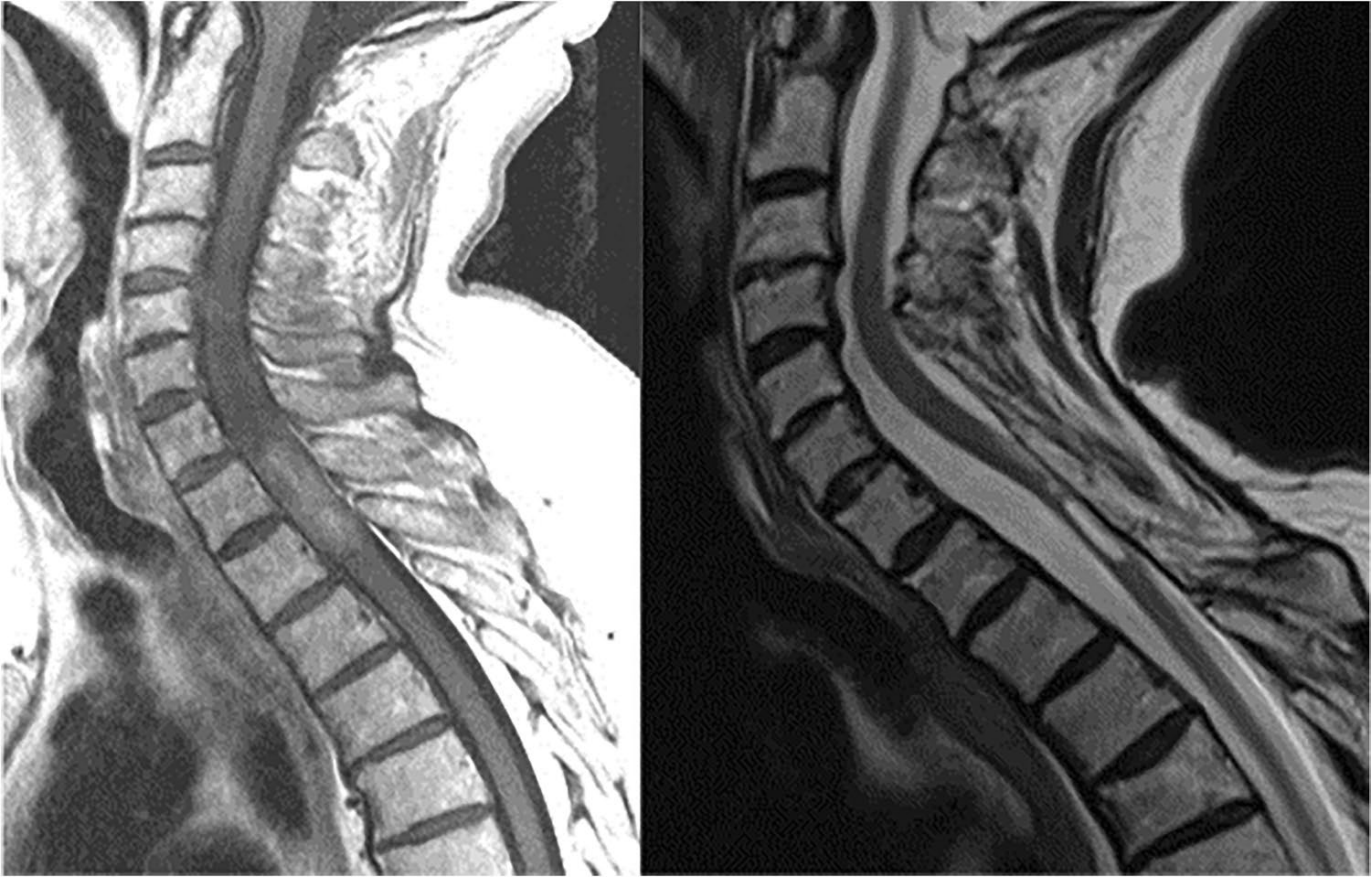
Ependymoma. MRI T1 preoperatively, T2 10 years postoperatively
